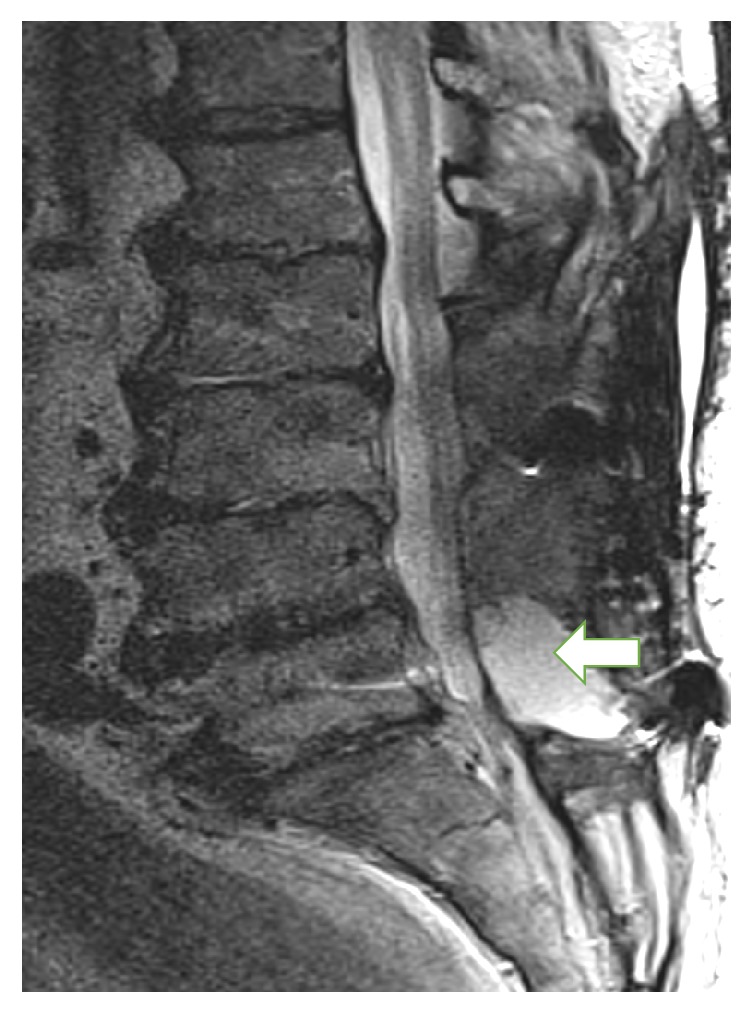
MRI from October 9; arrow designates a 3.5 cm fluid collection posterior to the thecal sac at the L5 level; aspiration proved sterile (T2-weighted image provided by Craig Sherman).
